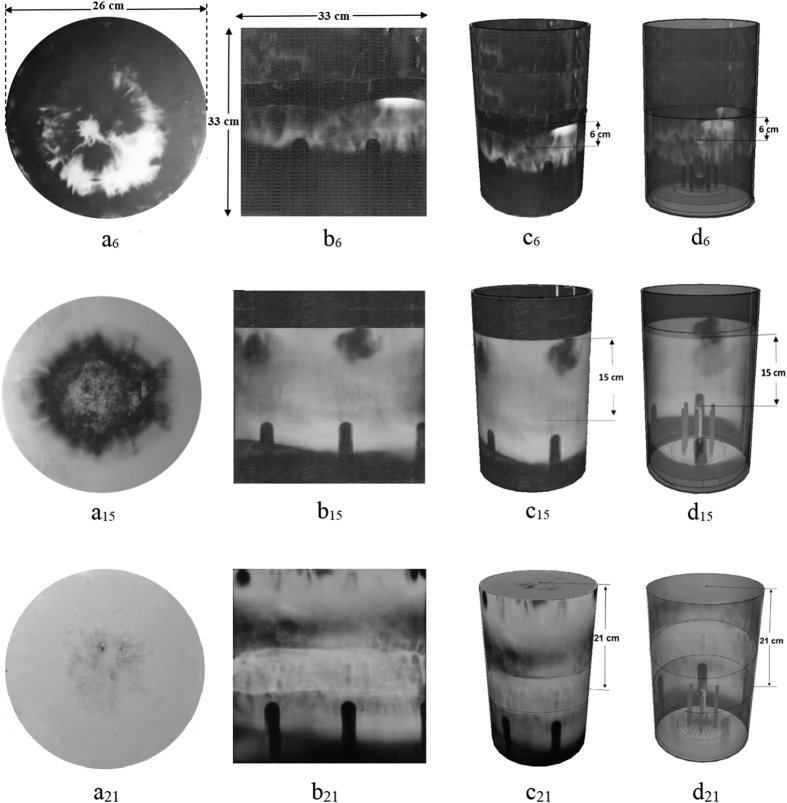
(a–d) 3D ion emission distribution images in 4π PFD space. (a_6,15,21_) Images of helium ion angular distributions on circular MSPC detectors at either 6, 15 or 21 cm distances from the “anode top”; (b_6,15,21_) images of helium ion angular distributions on half of a MSPC wall detector simultaneously exposed with a circular MSPC detector at a known distance; (c_6,15,21)_ images of helium ion distributions on circular and wall MSPC detectors when assembled together forming 3D ion distributions in PFD space by applying a SketchUp Software in a FaceStyle (shaded with shadows) mode at either 6, 15 or 21 cm distances; and (d_6,15,21_) images of ion emission angular distributions of circular and wall MSPC detectors when assembled forming 3D ion distributions in PFD space by applying a SketchUp Software in a FaceStyle (X-ray) mode at either 6, 15 or 21 cm distance.
